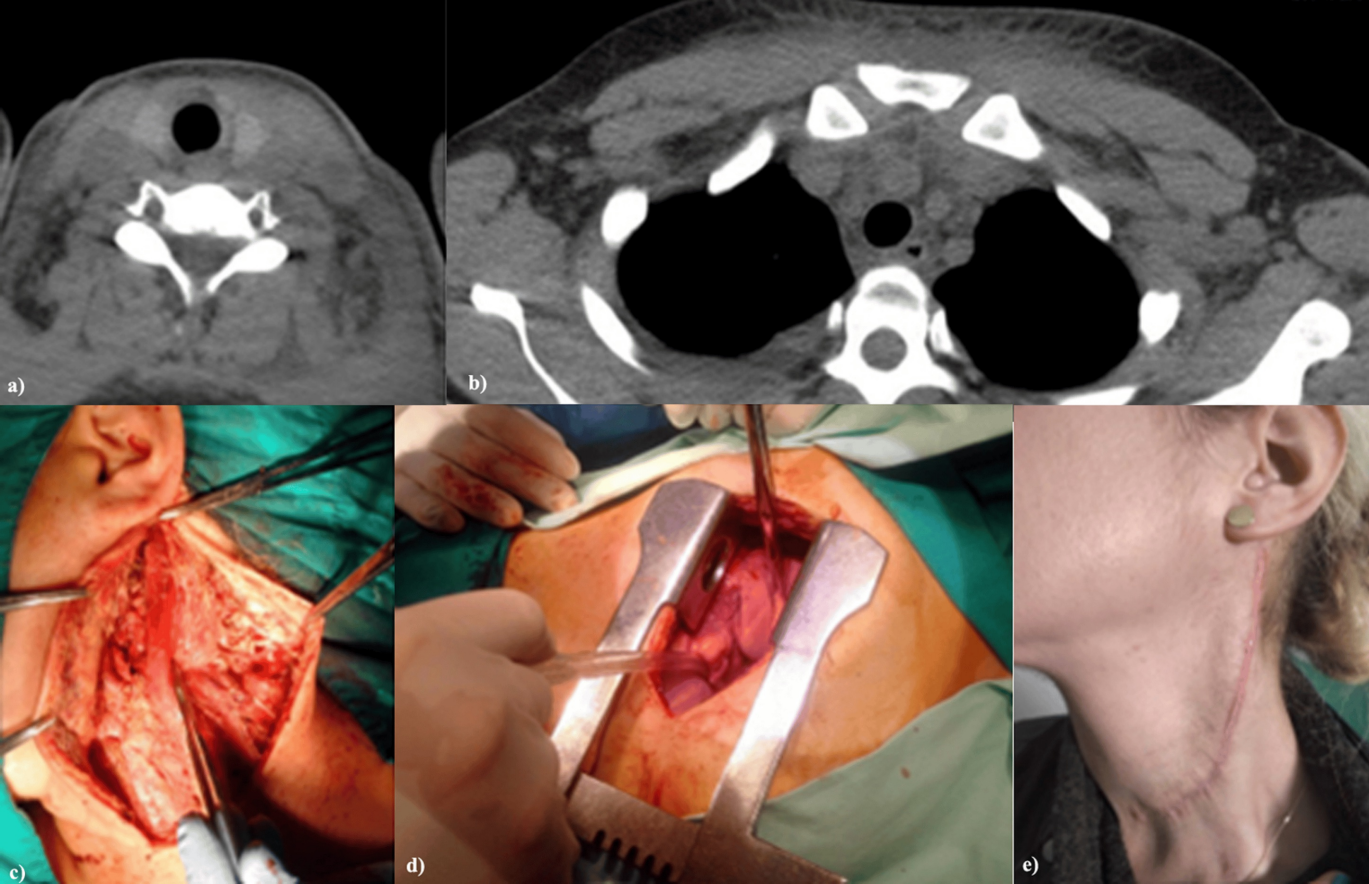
Cervical and thoracic CT appearance and intraoperative findings in a 32-year-old female patient with left cervical hematoma due to aggression, complicated with cervicomediastinitis. (a) Axial CT scan of the neck. (b) Axial CT scan of the thorax. (c) Surgical aspect from the neck. (d) Surgical aspect from the thorax. (e) Final aspect two weeks after discharge.
